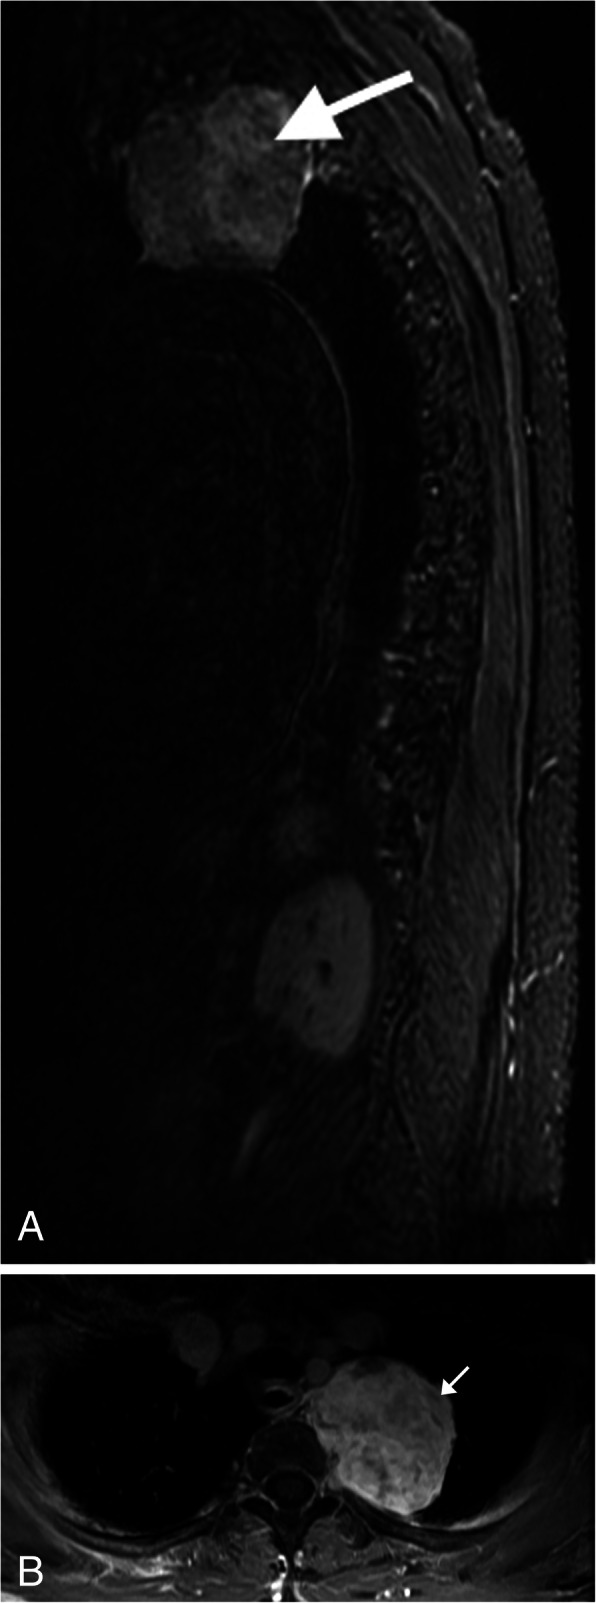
Schwannoma. a Sagital T2WI MR image demonstrates a well-circumscribed heterogeneous, predominantly hyperintense lesion arising from a right upper thoracic neural foramen. The lesion has multiple internal low-intensity ring-like structures (arrow), called the fascicular sign (representing fascicular bundles within the nerve). b Axial T1WI MR post contrast image of the thoracic spine in a companion case demonstrates heterogeneous enhancement of the lesion arising from the left T2 neuroforamina (arrow)
